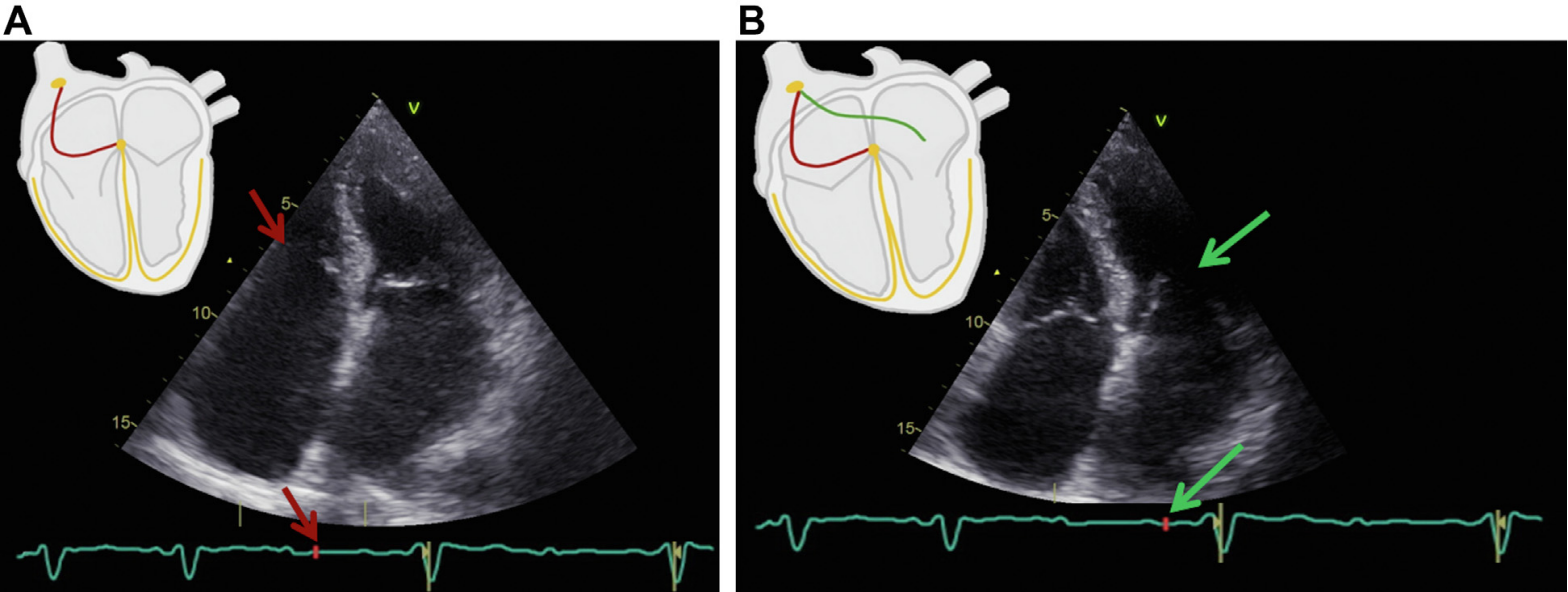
Transthoracic Echocardiography (A) Activation of the right atrium (red lines) with opening of the tricuspid valve and appearance of the first P-wave (red arrows). (B) Activation of the left atrium (green lines) with opening of the mitral valve (green arrows) and appearance of the second P-wave.
